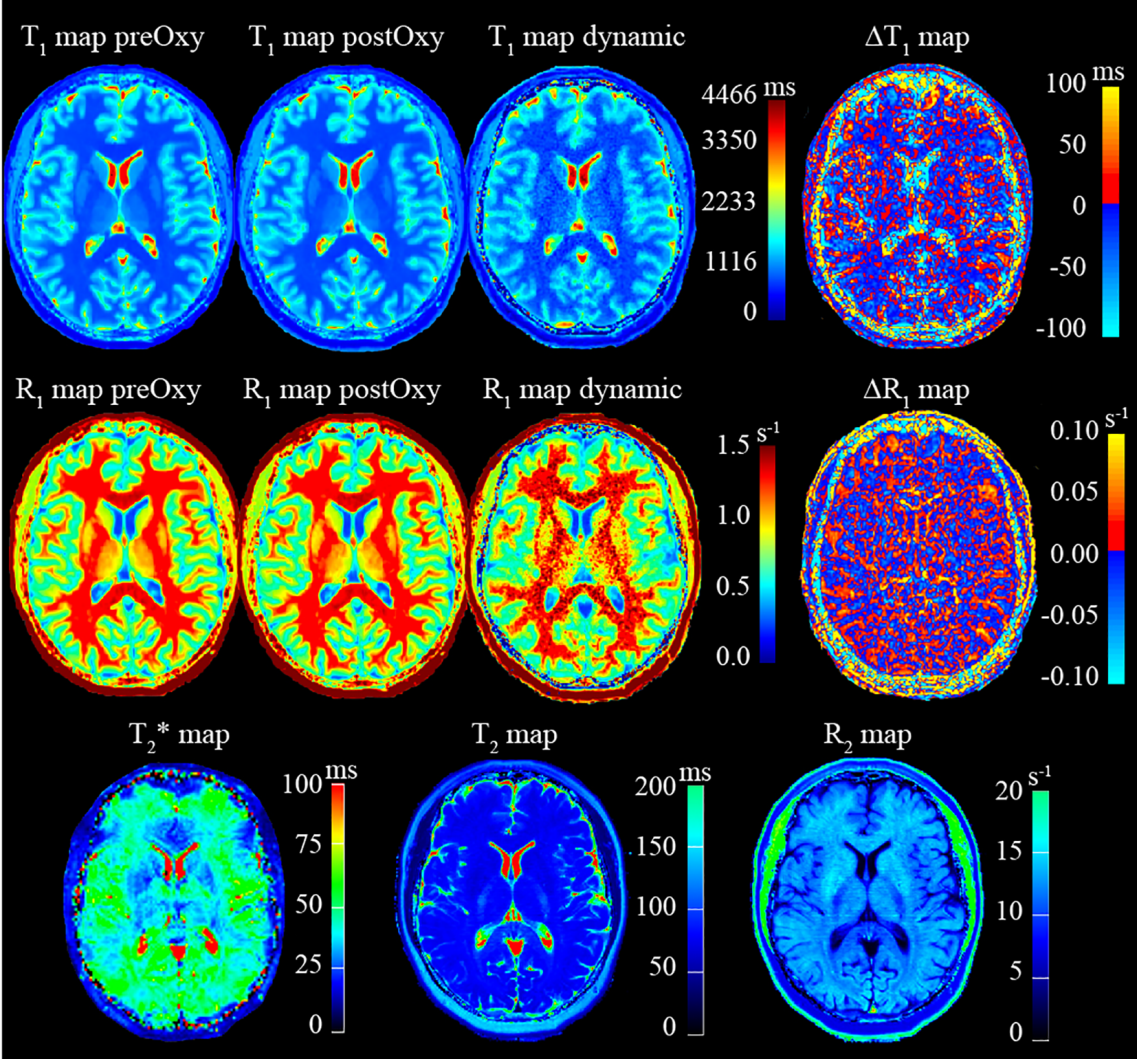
Example of quantitative images obtained from MRI protocol acquisition on a healthy volunteer.
